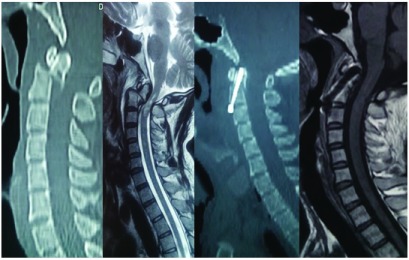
Image showing placement of the odontoid screw and the follow up CT and the MRI scans.
